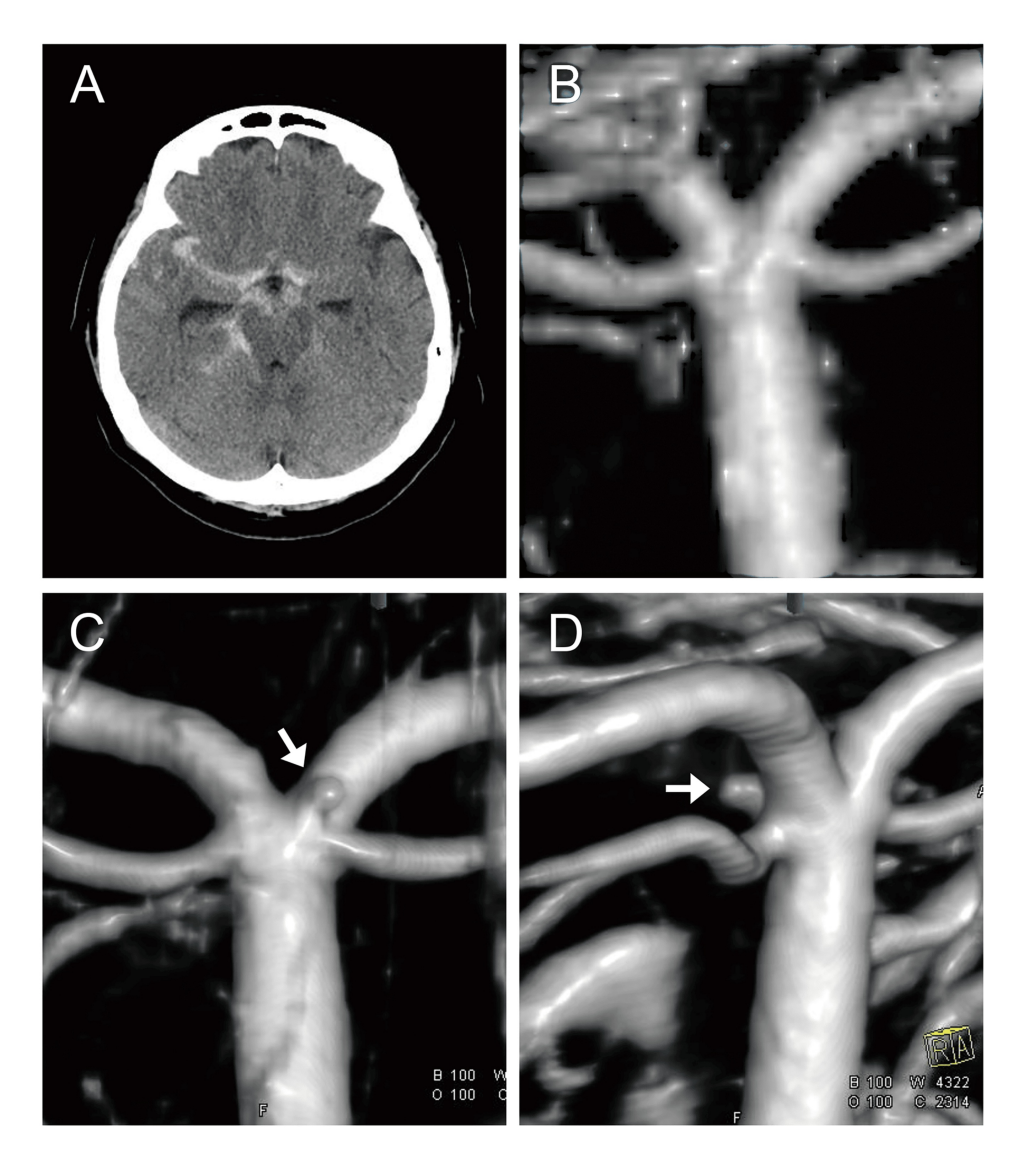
Imaging findings for the patient, a 74-year-old woman. A: Initial head CT scan showed a subarachnoid hemorrhage in the right prepontine cistern and right Sylvian fissure. B: 3D-DSA performed on day 2 showed no obvious aneurysm. C, D: 3D-DSA performed on day 14 showed a blood blister-like aneurysm on the posterior wall of the basilar artery tip (C: posterior view, D: right anterior oblique view). Arrows indicate the aneurysm. DSA: digital subtraction angiography.
